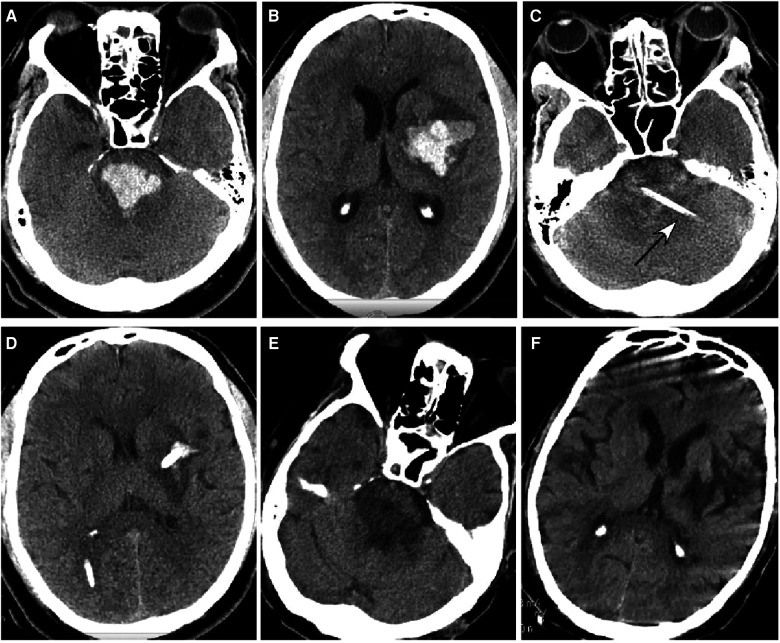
Brainstem hemorrhage. (A,B) A 45-year-old man had a sudden loss of consciousness 1 h before presentation. Head CT scan (A,B) showed severe hemorrhage in the brainstem, thalamus, and basal ganglia. The patient was in a coma with a Glasgow Coma Scale (GCS) score of 3 (E1VTM2). (C,D) An ultra-early stereotactic aspiration was performed (arrow). (E,F) Three months after surgery, the patient had a GCS score of 6 (E3VTM4) and was in active rehabilitation.
